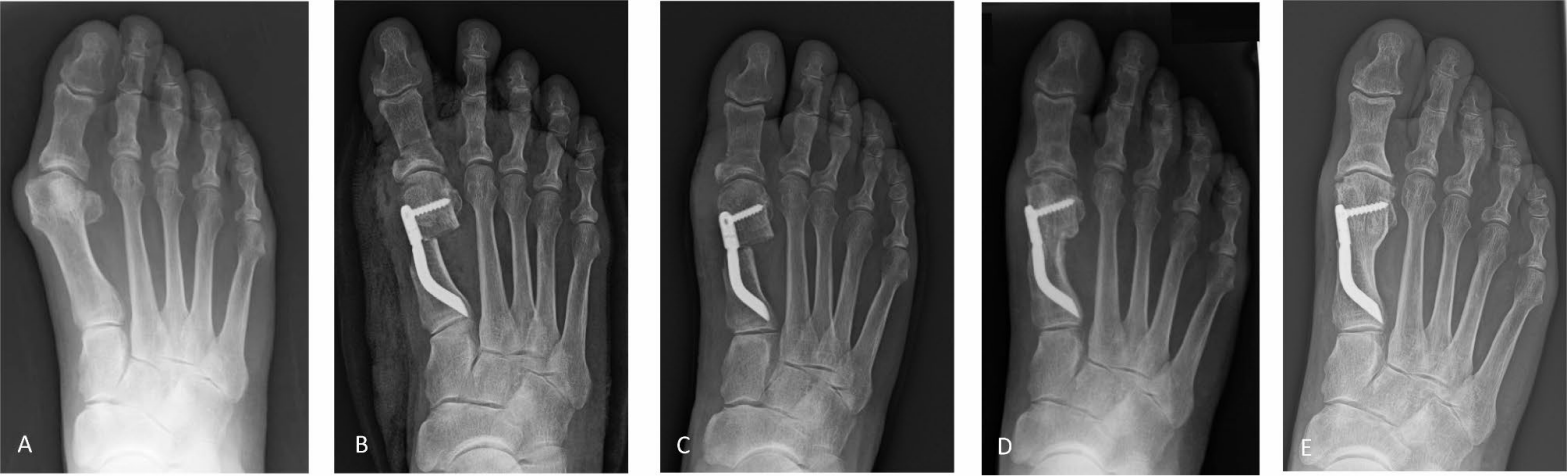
Case 2: A 58-year-old woman having undergone the Minimally Invasive Intramedullary Nail Device technique with Akin percutaneous osteotomy for severe HV correction of her right foot. Weightbearing radiographic images of anteroposterior view at (A) preoperative period, (B) 1-month follow-up, (C) 3-month follow-up, (D) 12-month follow-up, and (E) last follow-up at 60 months after surgery, showing bone callus consolidation and remodeling, a stable and long-lasting radiographic correction
